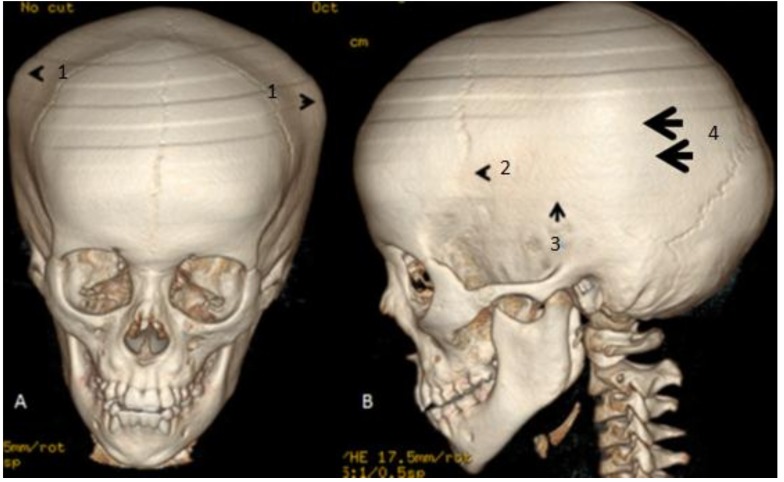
(A,B) 3D reconstruction CT scan of the cranium (anterior and lateral views) in the 17-year-old girl showed marked bulging of the frontal area associated with overlapping of the posterior cranial vault because of extreme bulging of the temporal bones (hat-like appearance in connection with extreme bulging of the skull bones over the temporal areas (arrowheads1). Partial closure of the coronal sutures (arrowhead 2) associated with total closure of the squamosal sutures (arrow 3) resulted in the development of bony bulging over the synostosed squamosal sutures. The bony bulge extended over the squamosal suture arches posteriorly from the pterion and connected the temporal squama with the inferior border of the parietal bone (hat-like appearance in the anterior view) (arrowheads 1) and (arrows 4) in the lateral view 4. Note the persistence of the metopic, sagittal, and lambdoid sutures (Figure 1A,B).
